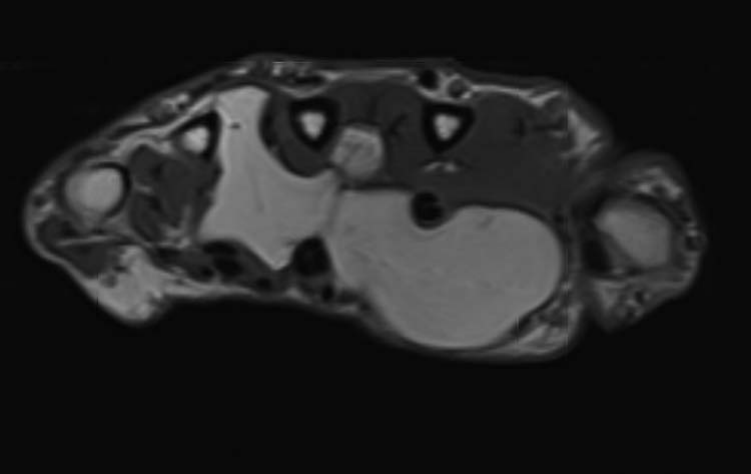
Axial MRI view demonstrating volar and dorsal extension of the fatty lesion from the middle of the palm.
